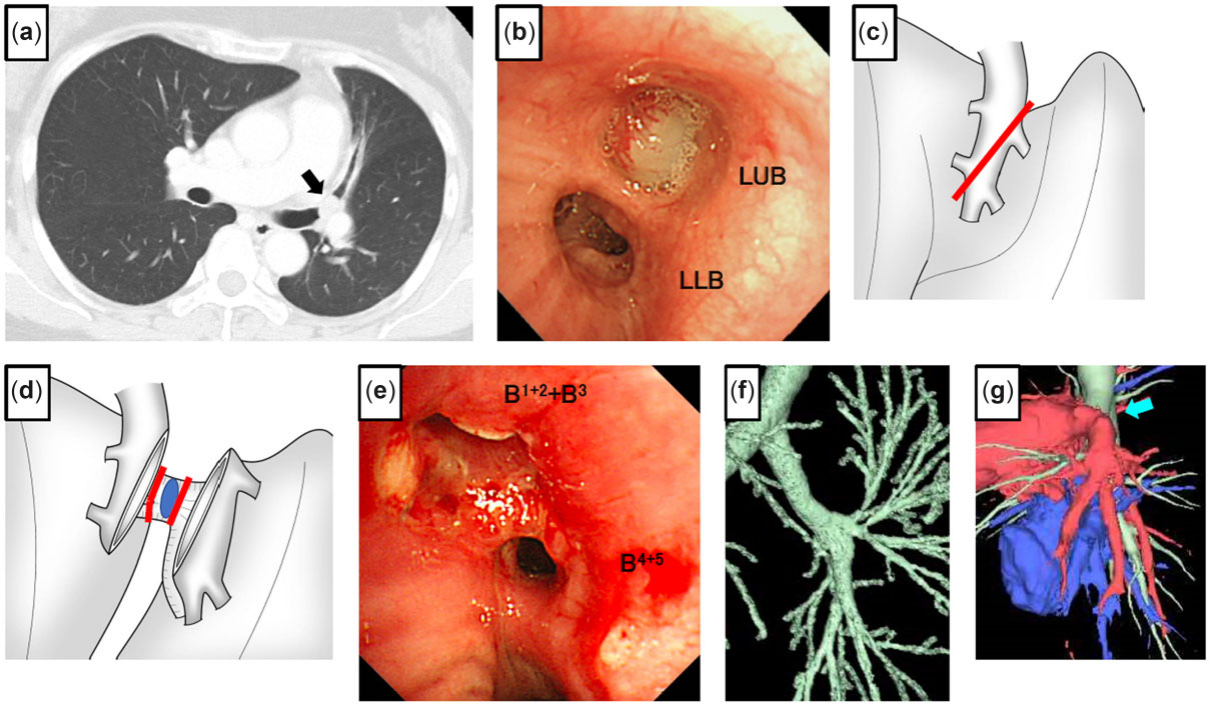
Chest computed tomography shows a tumour occupying the left upper bronchus (LUB); the arrow points to a tumour (a). Bronchoscopy before surgery shows a tumour obstructing the LUB (LLL: left lower bronchus) (b). The interlobar pulmonary artery was divided obliquely to expose the LUB (c). The LUB was exposed and removed with the tumour (d).
